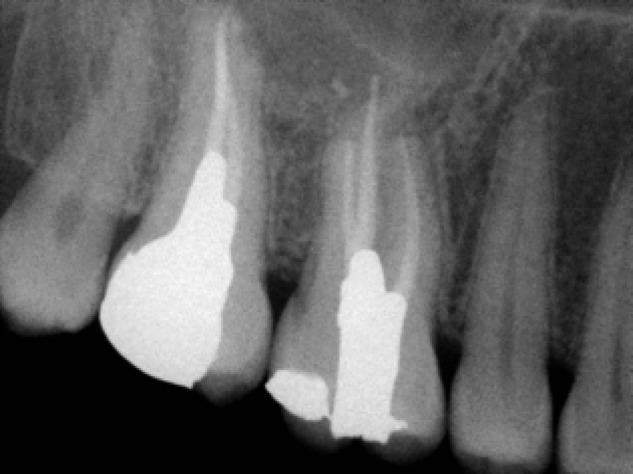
Periapical radio-graph demonstrating healing on the 12-months recall visit
